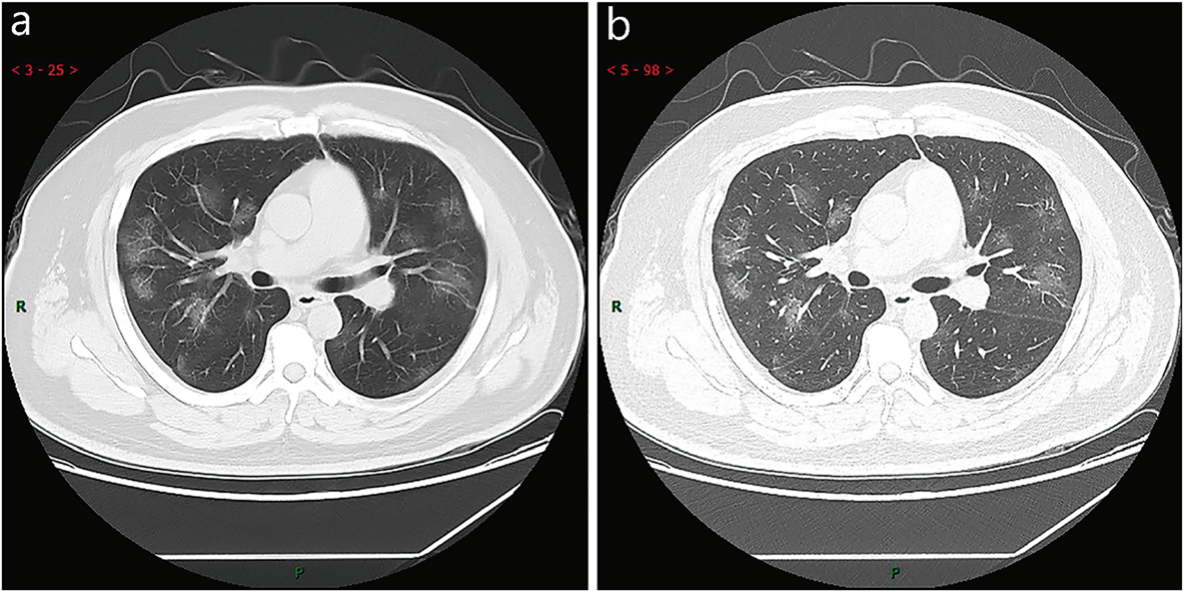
Typical CT imaging manifestation (case 1). A 38 years old male with fever without obvious inducement (39.3 ℃), dry cough and shortness of breath for 3 days. Laboratory test: normal white blood cells (6.35 × 10^9^/L), decreased lymphocytes percentage (4.1%), decreased lymphocytes count (0.31 × 10^9^/L), decreased eosinophil count (0 × 10^9^/L)), increased C-reaction protein (170.91 mg/L), increased procalcitonin (0.45 ng/ml). Imaging examination: multiple patches, grid-like lobule and thickening of interlobular septa, typical “paving stone-like” signs. a SL(Slice): 6 mm; b high-resolution computed tomography(HRCT). HRCT. high-resolution computed tomography
